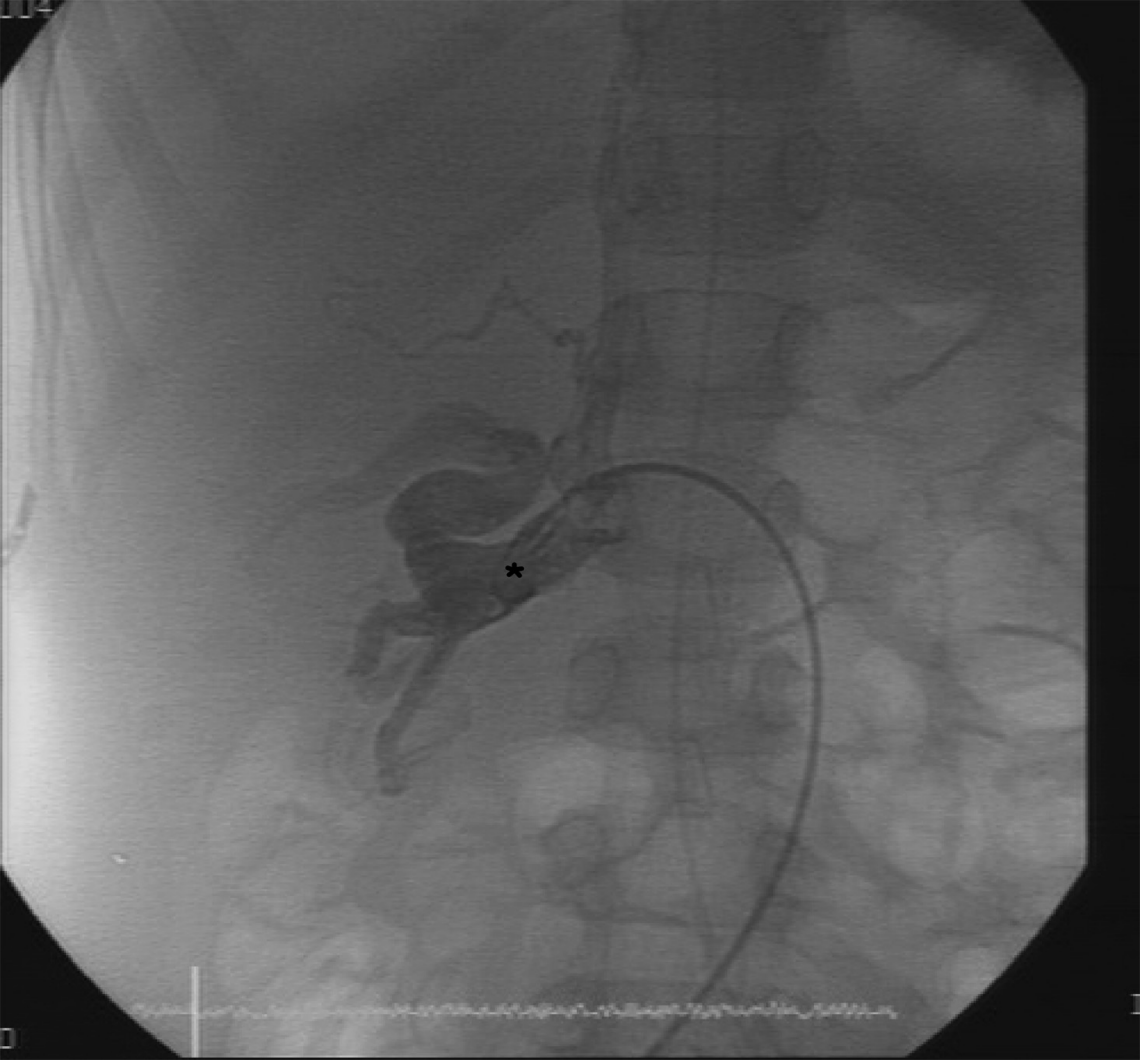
Azygos-portal vein collateral in a patient candidate for Fontan. X-ray angiography showing an Azygos-portal vein collateral (*) missed at MRA.
